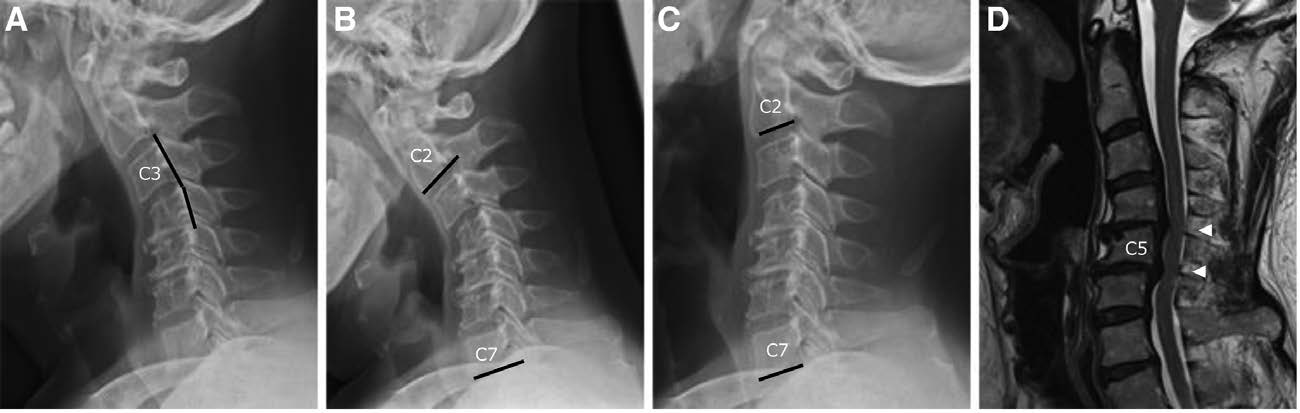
Preoperative images. (A) Neutral plain X-ray of the cervical spine. (B) Anteflexion position. (C) Retroflexion position. (D) MRI T2-weighted sagittal view.
